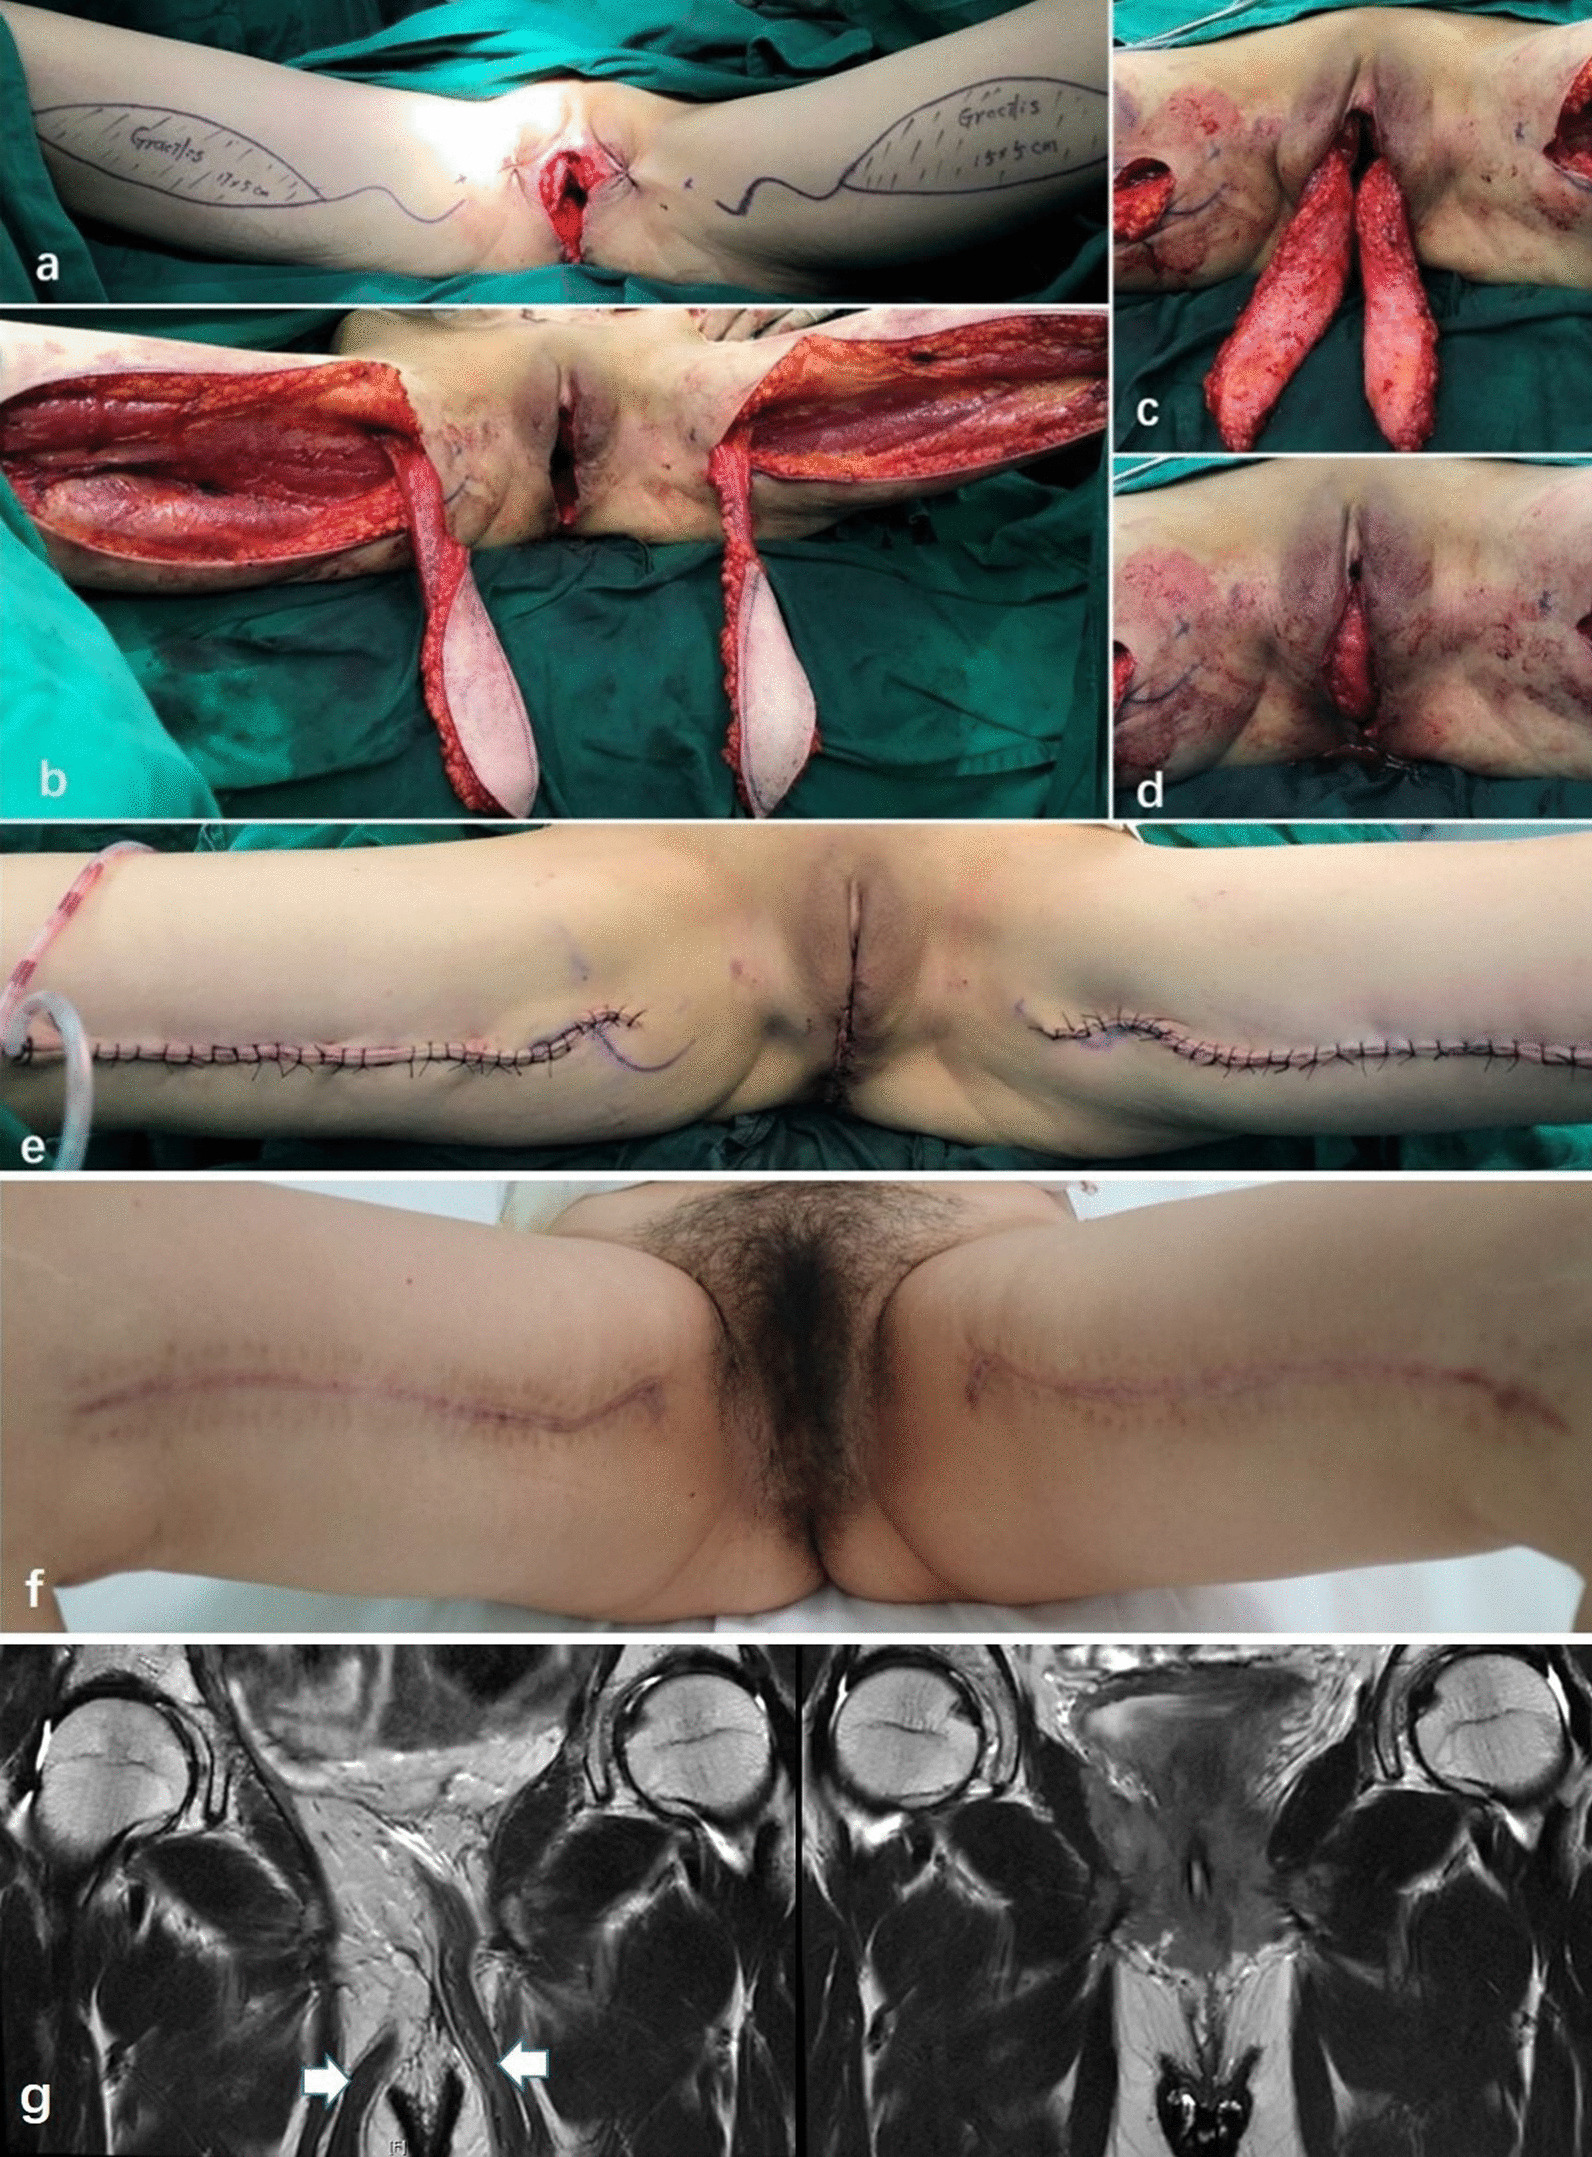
a Pelvic floor defect after pelvic exenteration and design of gracilis flaps on the inner side of both thighs. b The flap is freed with the proximal end of the muscle as a pedicle. c The skin was removed, and the muscle and subcutaneous tissues of the fascia were retained to prepare the gracilis adipofascial flap. The flap was rotated at 180° into the pelvic floor defect through the subcutaneous tunnel. d The pelvic floor defect was repaired with the gracilis adipofascial flap. e The recipient and donor sites were sutured. f 8 months postoperatively. g Gynecological MRI indicated good survival of the muscle flap, as marked by the white arrow (preoperative on the left side and postoperative 4 months on the right side)
